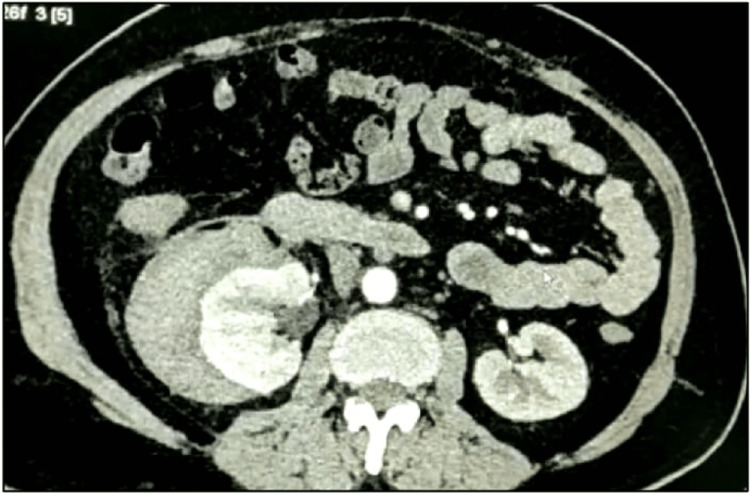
Computed tomogram showing the right kidney compressed medially by hematoma formation
